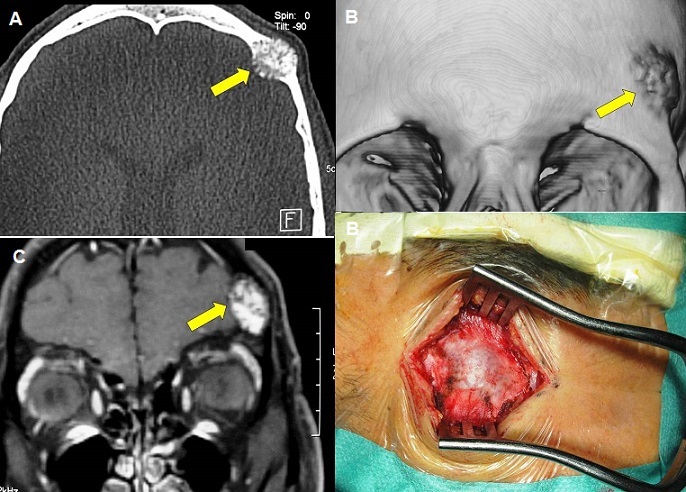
Cranial CT-scan on axial view (bony window) (A) and 3-D bony reconstruction (B) showing an osteolytic intradiploic lesion with poorly defined margins in the left frontal sus-orbital region. Coronal T1-weighted MRI after gadolinium administration showing the hyperintense extradural lesion with slight brain mass effect (C). Operative view of the bony bluish lesion before resection (D)
